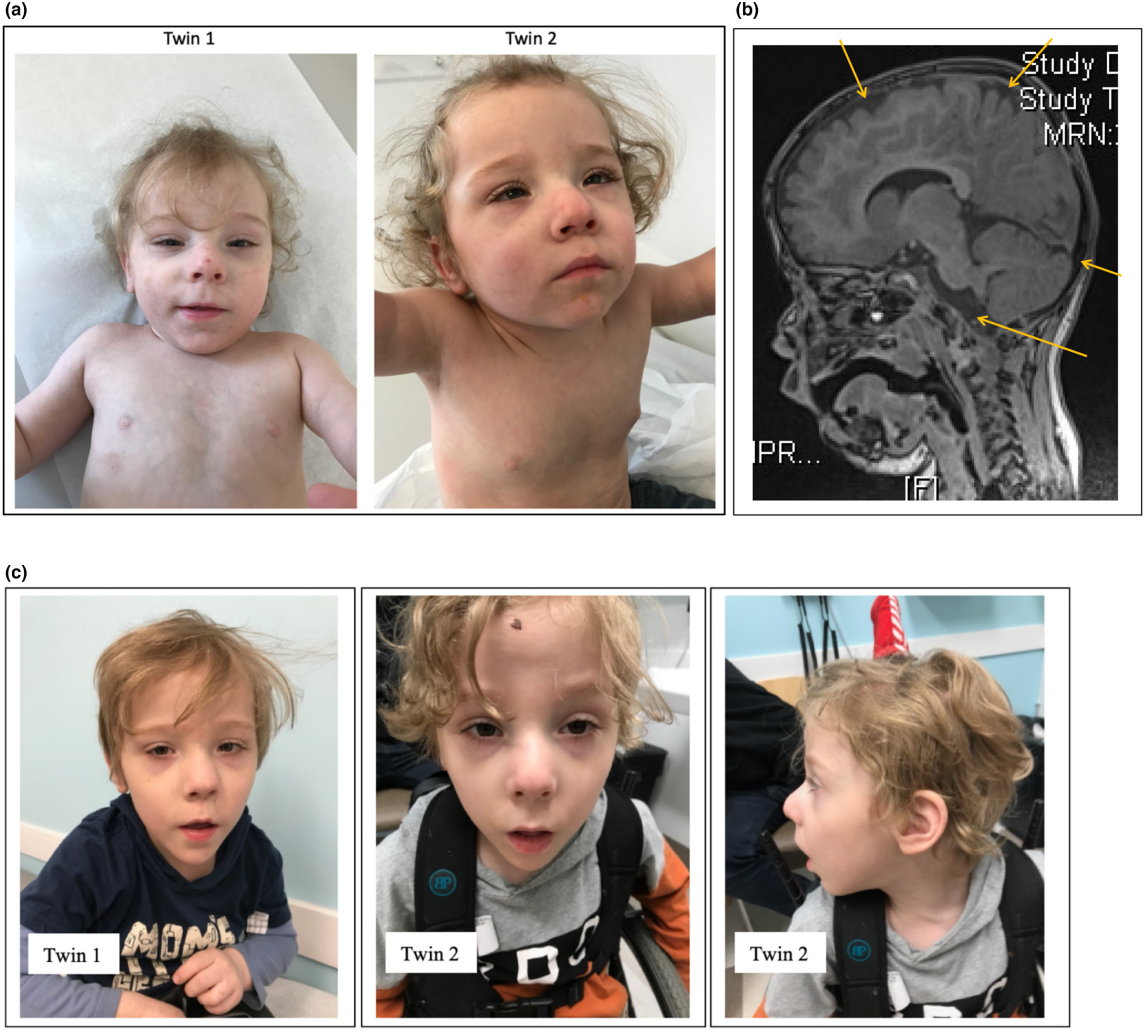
(a). Facial dysmorphism in twins with 20p13 duplication. Note thin hair, deep‐set eyes, hypertelorism, narrow palpebral fissures, long eyelashes, broad nasal bridge; bulbous nose, and long philtrum. Age: 2 years, 2 months. (b) T1‐weighted midline sagittal image in Twin 1. Mild nonspecific prominence of supratentorial sulci and cisterns consistent with mild cerebral volume loss (arrows). Minimal increased FLAIR signal consistent with periventricular gliosis or leukomalacia. (c) Dysmorphic facial features in twins with 20p13 duplication syndrome, at 5 years of age. Note triangular facies, in comparison to younger age.
